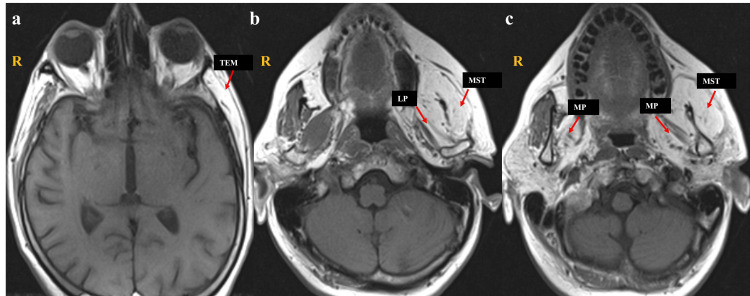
Axial T1-Weighted MRI Demonstrating Chronic Denervation-Related Fatty Atrophy of the Muscles of Mastication Axial T1-weighted non-fat-saturated MR images obtained at the level of the temporalis (a), lateral pterygoid (b), and medial pterygoid (c) muscles demonstrate marked volume loss and near-complete fatty replacement involving the left temporalis (TEM), masseter (MST), lateral pterygoid (LP), and medial pterygoid (MP) muscles, consistent with chronic denervation changes. Fatty infiltration and mild volume loss are also noted within the right medial pterygoid muscle. R = right side.
